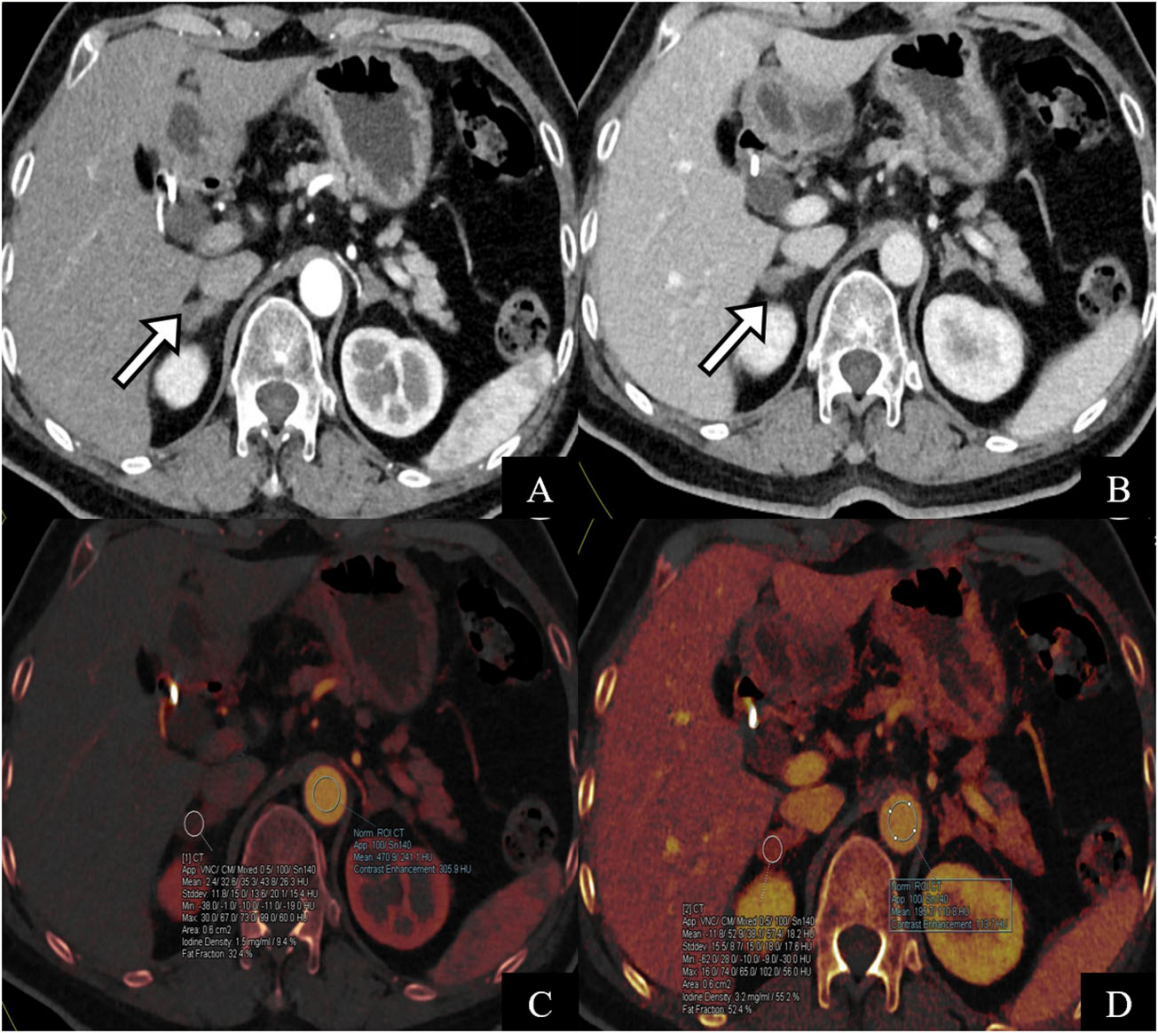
A 64-year-old female patient with surgically pathologically confirmed of primary hyperaldosteronism. The CT scan of the adrenal glands showed soft tissue density of nodular adrenal glands bilaterally (A, B) pointed by white arrow). AVS indicated high secretion of aldosterone on the right side. (C, D) are iodine maps of the arterial phase. The iodine concentration in the arterial and venous phases was 1.7 mg/ml and 0.61 mg/ml, respectively, and the ICD was 1.09 mg/ml, which is consistent with the diagnosis of the right dominant side of AVS.
